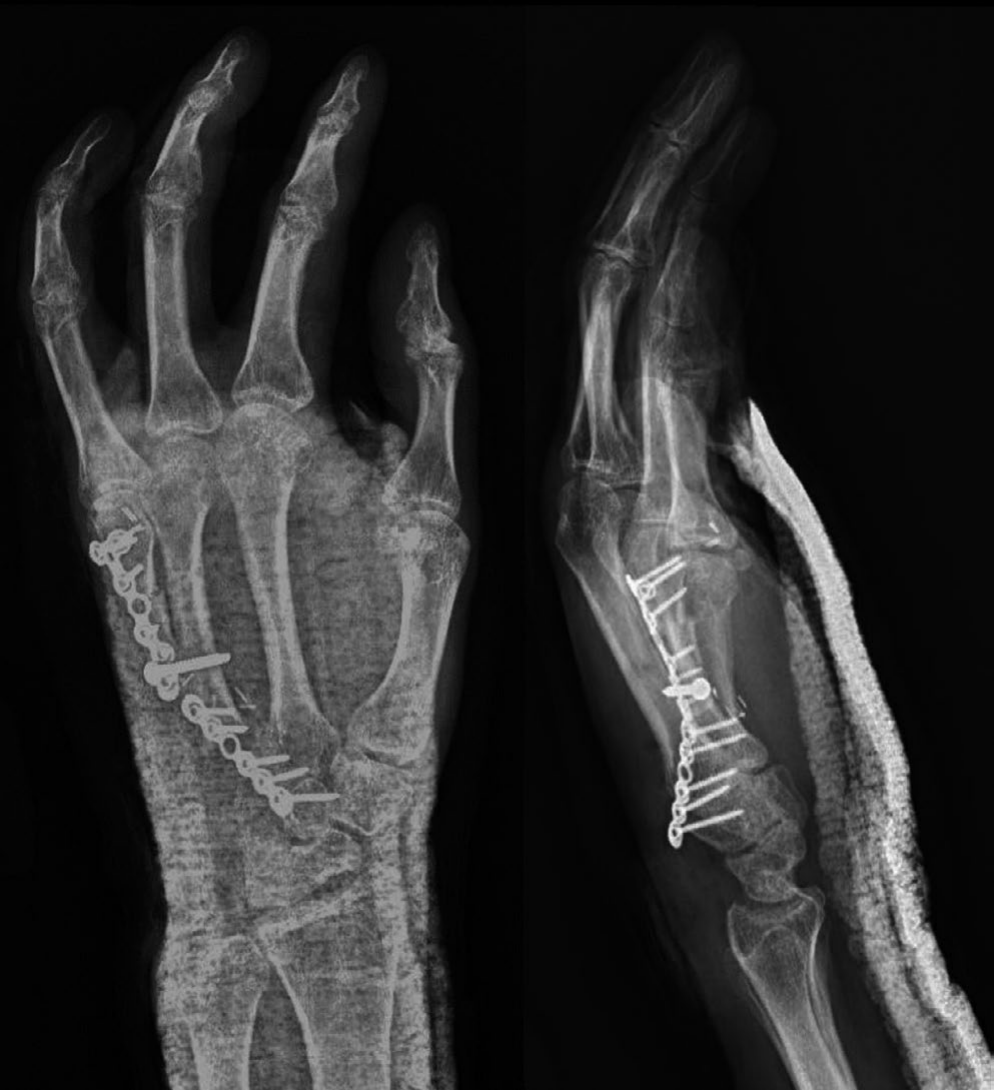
Intraoperative radiographs taken 20 months after the initial resection procedure
